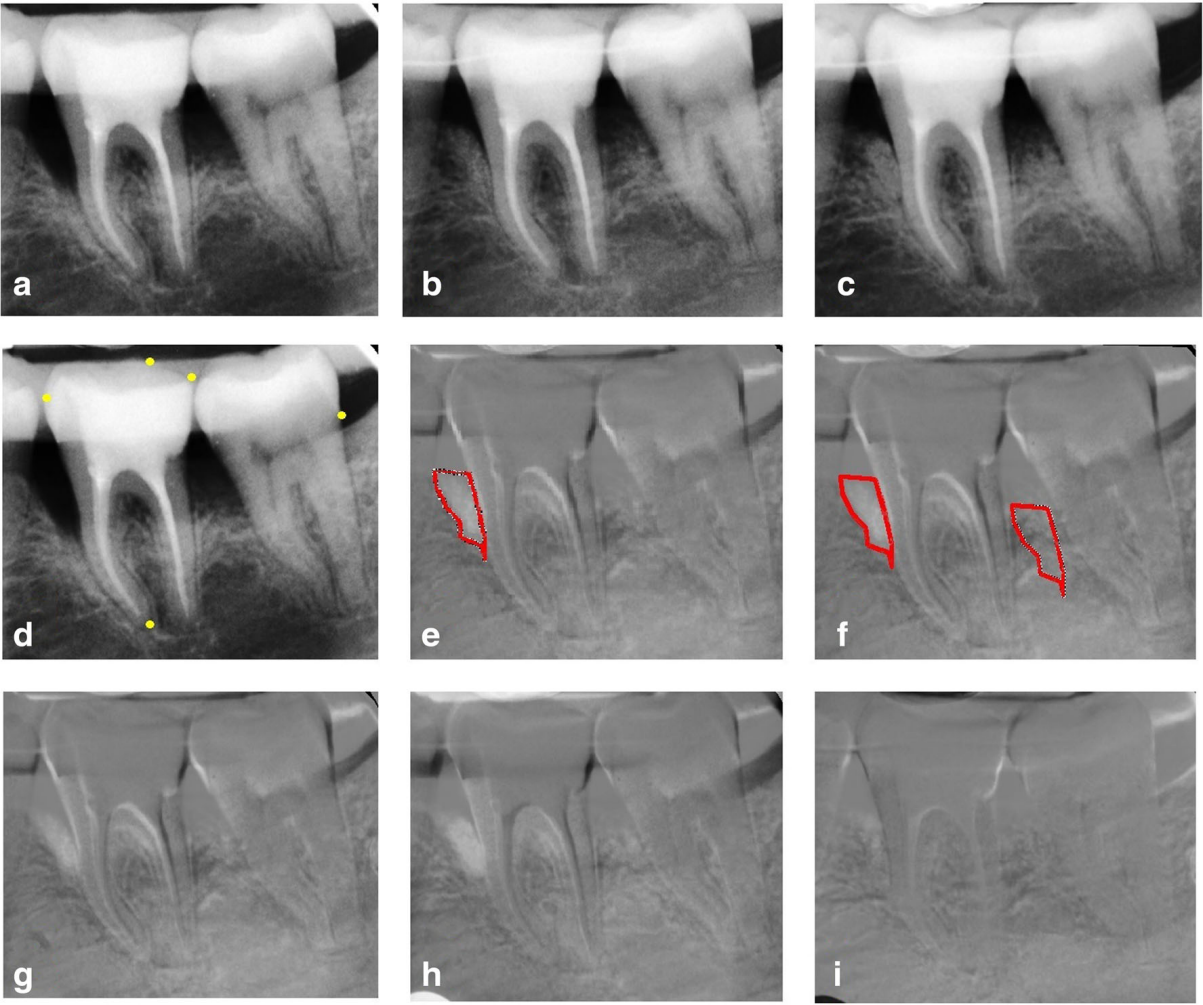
Subtraction radiography of test site (MPM-treated). Intrabony defect on mesial surface of tooth 36. a–d Radiographs after normalization. a Baseline radiograph. b Six-month radiograph. c Twelve-month radiograph. d Fixed points were marked (5 points) on baseline radiograph. e–i Subtraction images with a brighter area in the region of interest (ROI) representing the region with gain in density. e ROI was outlined by an irregular line corresponding to the borders of intrabony defect. f The reference ROI of the same shape was drawn on the healthy interradicular space as the region of control. g Subtraction of 6-month radiograph from baseline radiograph. h Subtraction of 12-month radiograph from baseline radiograph. i Subtraction of 12-month radiograph from 6-month radiograph
